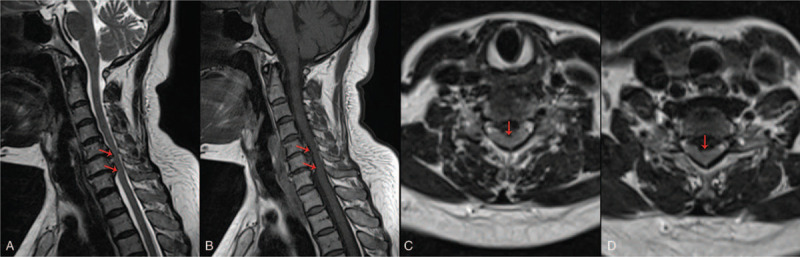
MRI showed spinal stenosis at the C5-C7 segment, C5-C6 and C6-7 cervical disc herniation and compression of the dura (red arrow). Besides, there were no abnormal signals in the cervical spinal cord.
